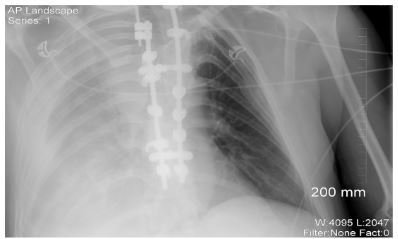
Portable chest radiograph showing significant right-sided extrapleural fluid.
